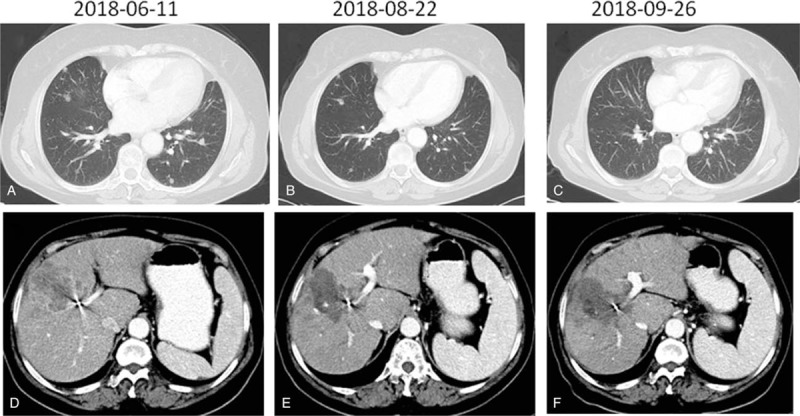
Computed tomography scan showed coexisting chest and abdominal masses (A and D) before treatment with anlotinib and microwave ablation. The sizes of the lesions in the chest and abdomen decreased significantly after combined treatment (B, C, E, and F).
